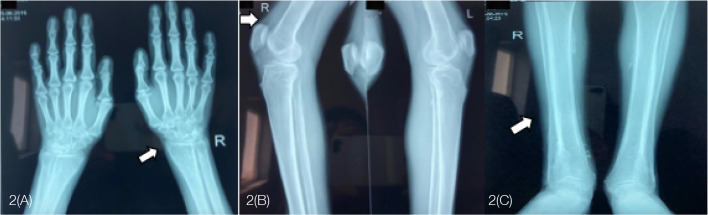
Radiograph of hand demonstrating widening of distal end of radius and ulna (white arrow). Figure 2(B). Radiograph of knee depicting irregular cortical thickening of bilateral femur (white arrow). Figure 2(C). Radiograph of legs showing sub-periosteal bone formation (white arrow).
